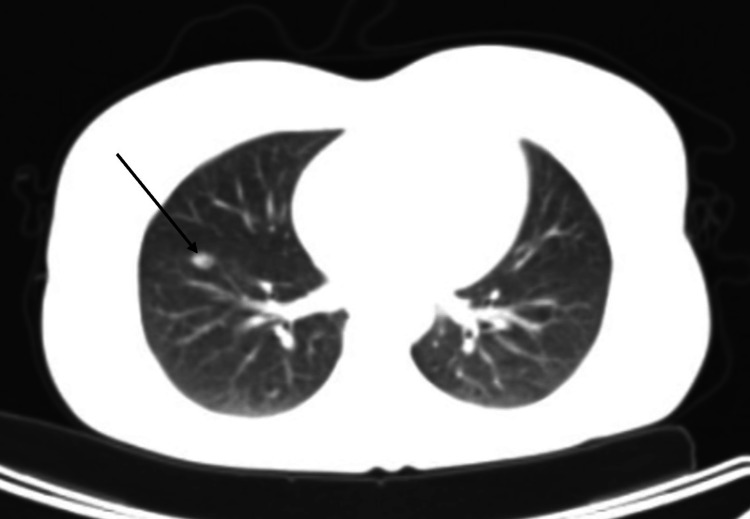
CT scan showing a prominent nodular lesion indicative of metastatic foci (black arrow)
